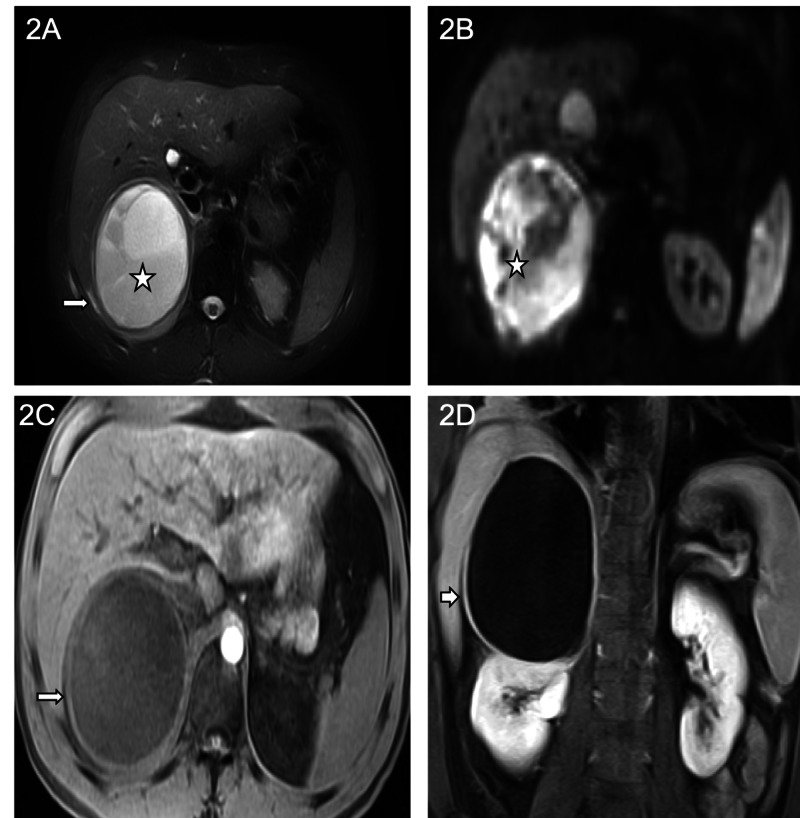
MRI Abdomen Axial T2 fat saturation, pre-contrast T1 weighted (T1 W), post-contrast T1W, and diffusion-weighted imaging (DWI) images, respectively, demonstrate a large T2 bright cystic mass (star) with a rim of edema surrounding the mass (arrow) (2a). On the DWI B500 (2b), there is marked restricted diffusion (star) in the entire cystic mass supporting an abscess. On pre and post-contrast T1W images (2c, 2d), there is a smooth enhancement of the wall (arrow). Notice there is no nodularity of the wall.
